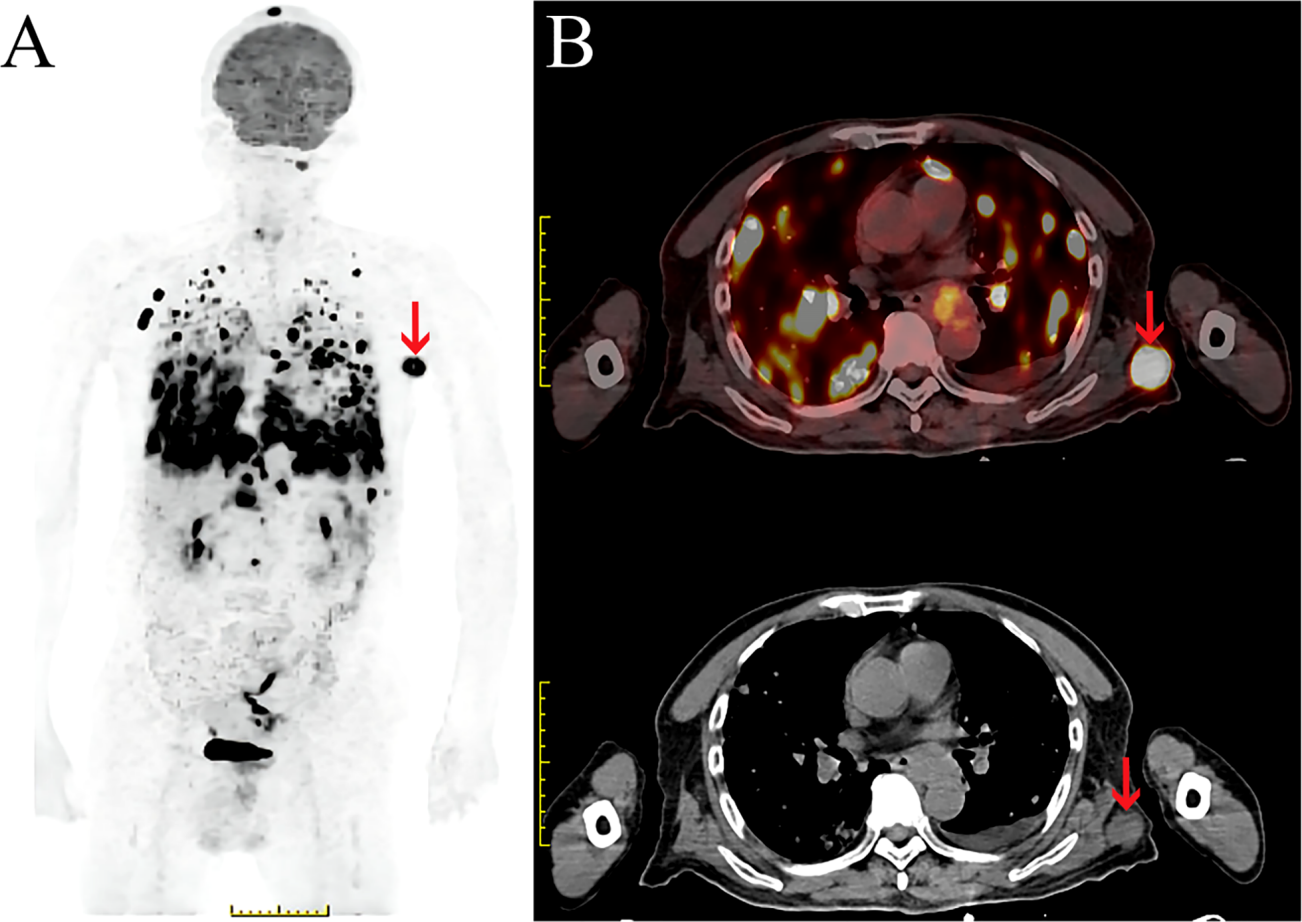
PET/CT scan of subcutaneous soft tissue metastasis in the left posterior thorax. (A, B). (A) Maximum intensity projection (MIP) image of the whole body demonstrates a large FDG avid nodule in the left thorax (red arrow). (B) Fused PET/CT and CT axial image demonstrates a large FDG avid nodule in the left posterior thorax (SUVmax=20.3) (red arrow).
